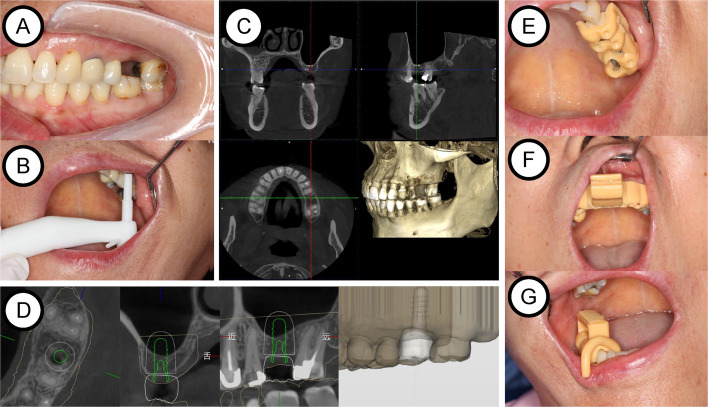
Preoperative preparation and data acquisition. A Intraoral view of the edentulous site. B Assessment of mouth opening. C Preoperative CBCT with multiplanar and 3D reconstruction. D Prosthetically driven implant planning in DentalNavi 3.0. E Intraoral try-in of the attachment for the posterior edentulous region. F Intraoral try-in of the attachment for the anterior region. G Mouth opener and suction tubing
